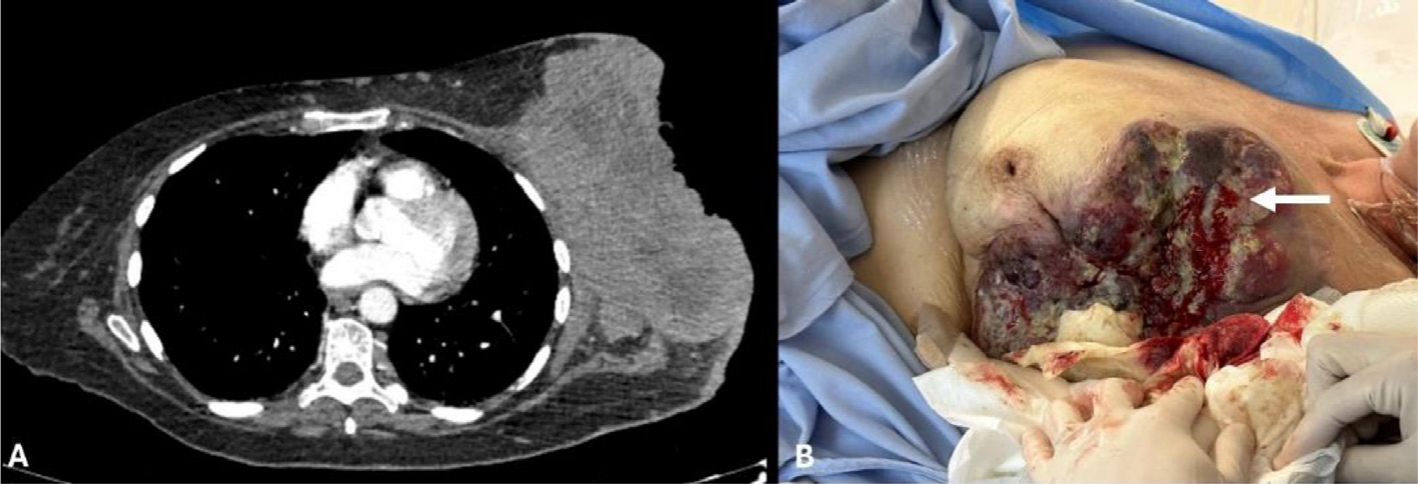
(A) CT scan showing the voluminous left breast mass. (B) Intraprocedural view showing the persistence of moderate bleeding (arrow) after embolization of the internal thoracic artery. This bleeding stopped after embolization of the axillary artery branches.
